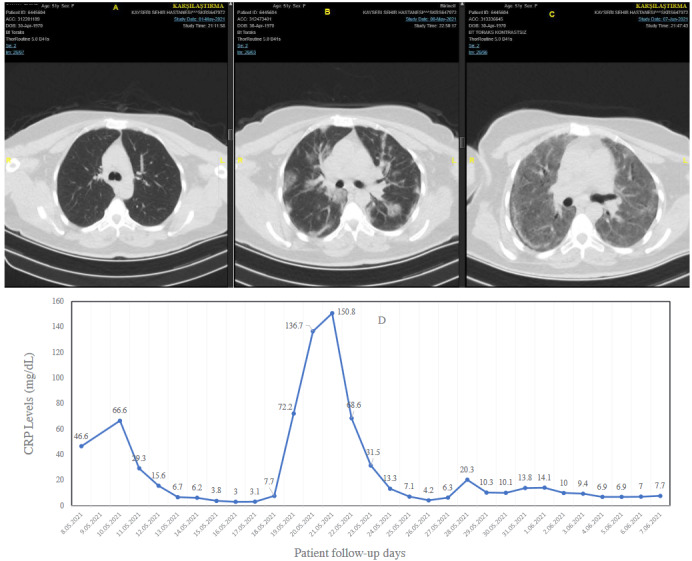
A sample for critical disease associated with COVID-19. Thoracic CT imaging and CRP course for 51-year-old women COVID19 patient with previously undiagnosed type II diabetes mellitus. A) Thoracic computed tomography at admission. B) Thoracic CT imaging on day eight. C) Thoracic CT imaging on the 37th day. D) Patient’s CRP course. Low-dose tocilizumab (100 mg, 100 mg, every other day at first CRP peak, and 80 mg, and 80 mg one dose at second CRP peak, every other day) four times (a total of 360 mg) and 250 mg three times for 3 consecutive days methylprednisolone and 40 mg/day for 20 days had been administered.
